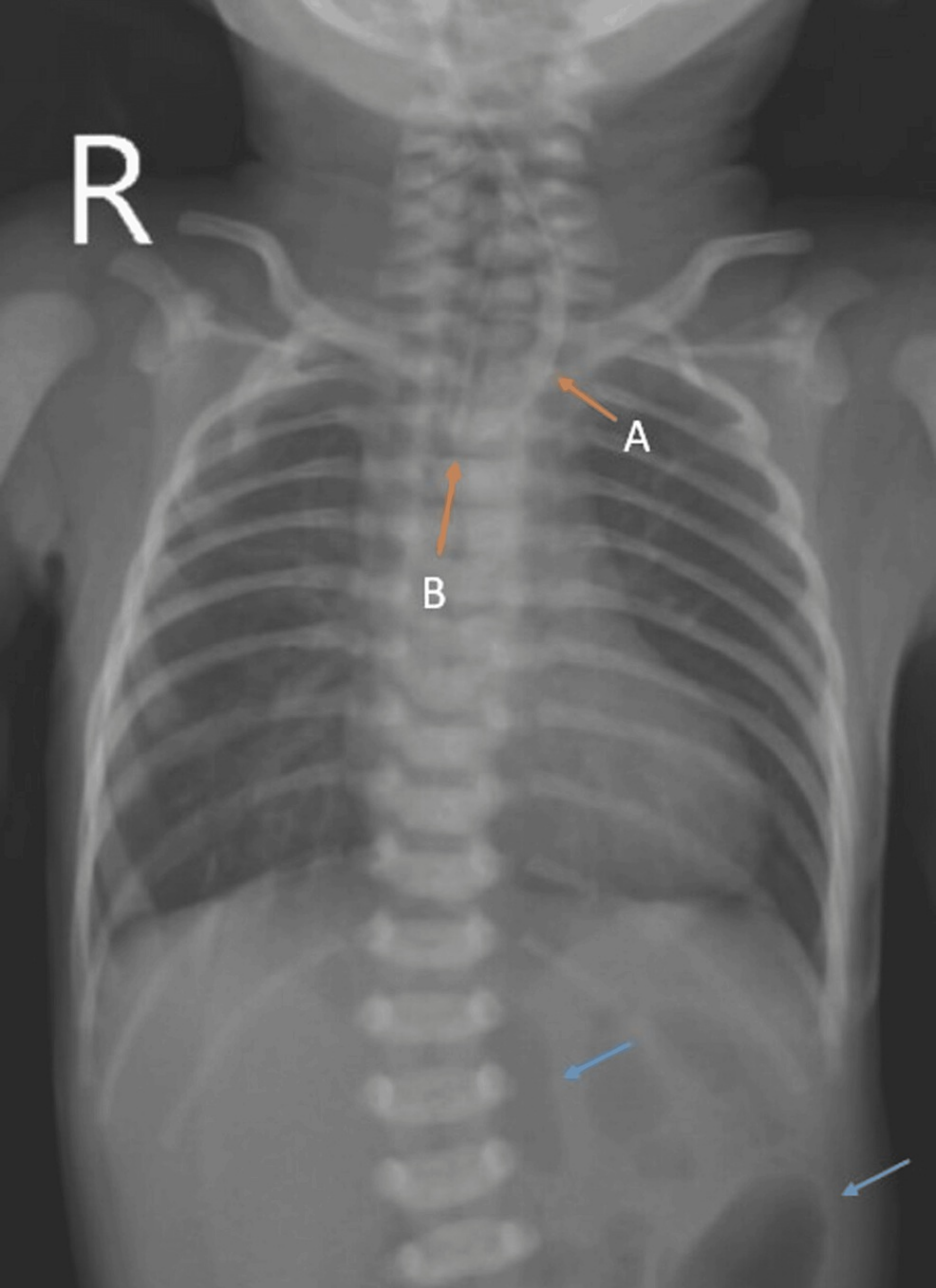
Chest X-Ray of the patient showing (A) nasogastric tube in the esophagus, and (B) coiling of the nasogastric tube within the esophagus (orange arrows) with presence of gas in the bowel (blue arrows) suggestive of proximal esophageal atresia with distal tracheoesophageal fistula.
